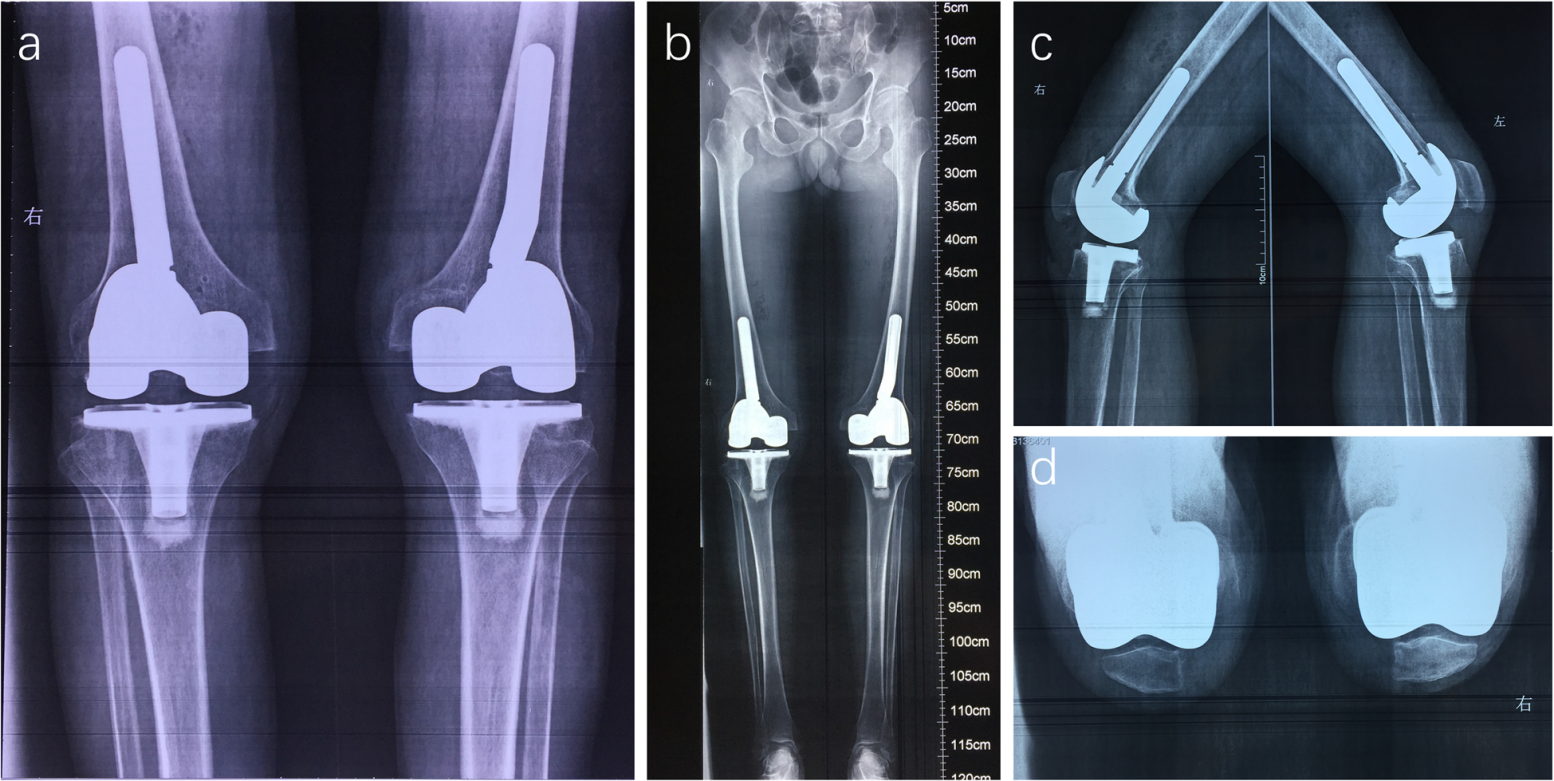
Postoperative plain radiographs. c Anteroposterior radiographs of both knees. b Anteroposterior radiographs of the full lower extremity. c Lateral radiographs of both knees. d Patellar axis radiographs of both knees
